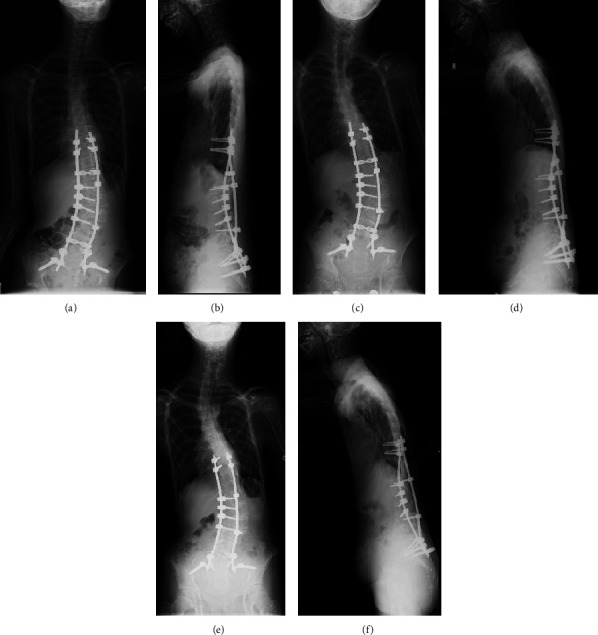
(a) Postoperative X-ray—AP view, levoscoliosis (T10–L5: 43°); (b) postoperative X-ray—lateral view; (c) rod breakage X-ray—AP view; (d) rod breakage X-ray—lateral view; (e) last follow-up X-ray—AP view; (f) last follow-up X-ray—lateral view.
